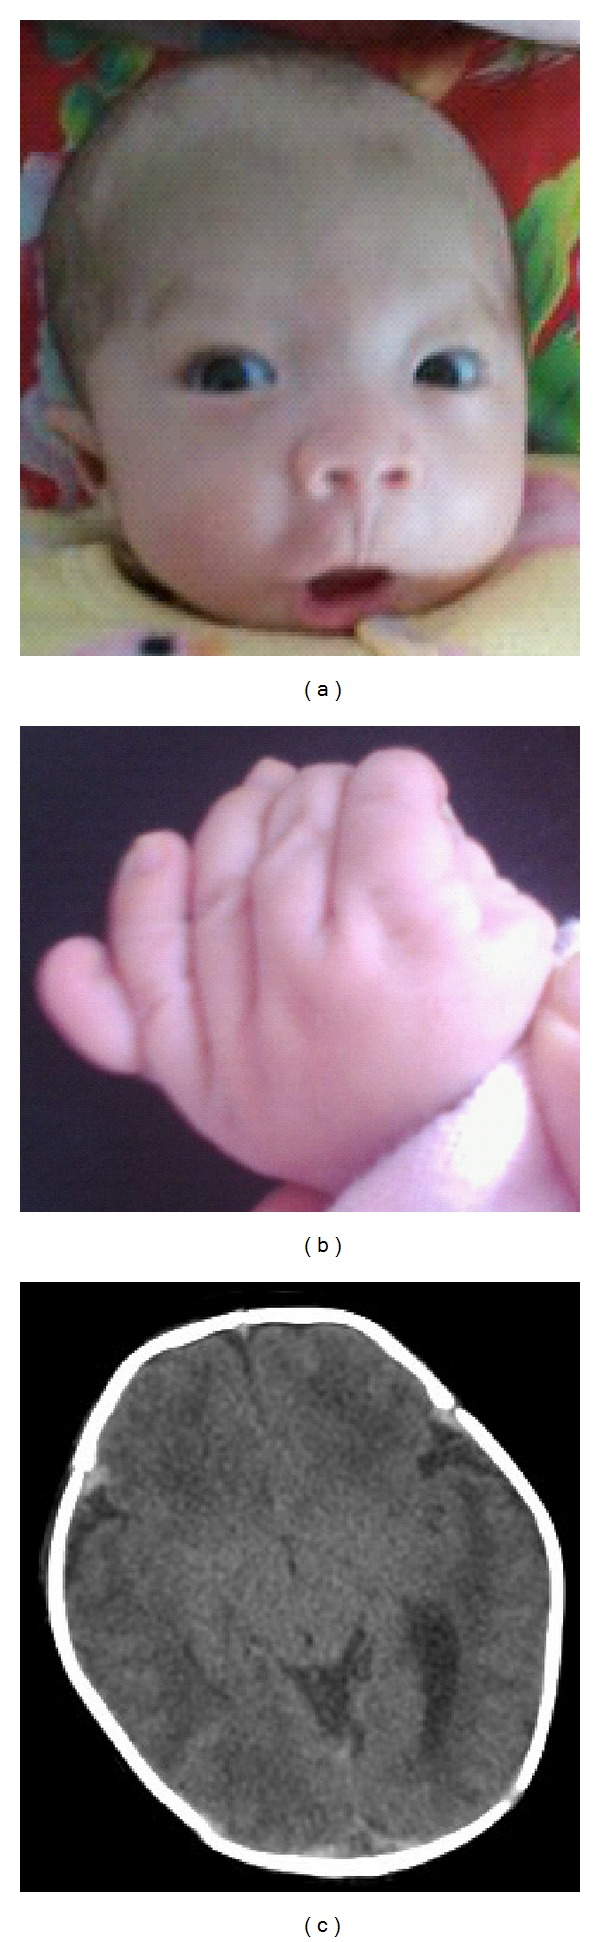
Photographs of patient at one-month old. (a) Facial features of frontal bossing, low and flat nasal bridge, low-set ears, nasal bridge hypoplasia, hypertelorism, and bilateral epicanthus. (b) Polydactyly of her left hand. (c) CT scan of subarachnoid hemorrhage.
